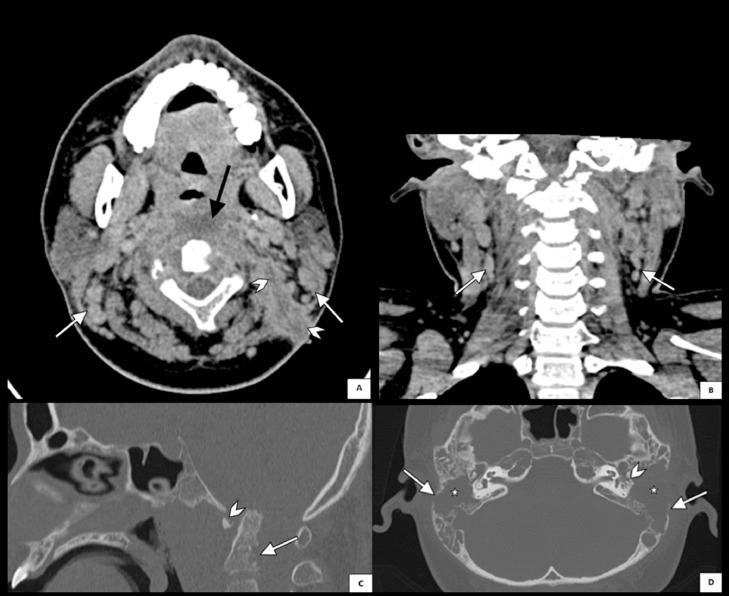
(A) Axial computed tomography (CT) scan of the brain shows an abscess with prevertebral involvement (black arrow) extending to the retropharyngeal and lateral wall with fistula formation (arrowheads), and enlargement of multiple bilateral level II-III cervical lymph nodes (white arrows). (B) Coronal CT scan of the neck also reveals enlargement of bilateral lymph nodes surrounding the neck (white arrows). (C) Sagittal CT scan of the temporal bone with bone window displays erosion of the clivus (arrowhead) and the C1-C2 vertebrae (white arrow). (D) High-resolution CT scan of the mastoid shows destruction of bilateral mastoid air cells opacity (stars) and erosion of the temporal bone with bony sequester and irregular borders (white arrows), with more prominent destruction of the left ossicles (arrowhead) suggesting tuberculous otomastoiditis.
